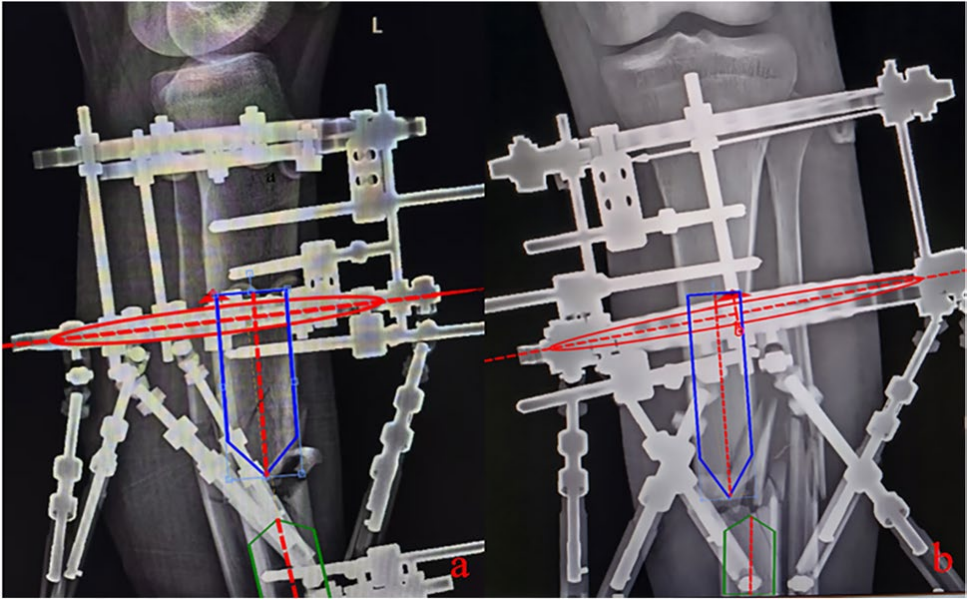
X-ray measurement of hexapod external fixator mounting parameters. a The elliptical and arrow markers were aligned with the corresponding rings and anatomical markings on the AP tibial X-ray images. b The elliptical and arrow markers were aligned with the corresponding rings and anatomical markings on the lateral tibial X-ray images
